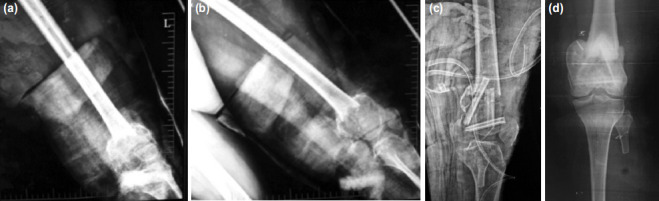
(a,b) Giant cell tumour of distal femur presenting with a pathological fracture. (c) Immediate post-operative radiograph. (d) Two years post-operative radiograph.
